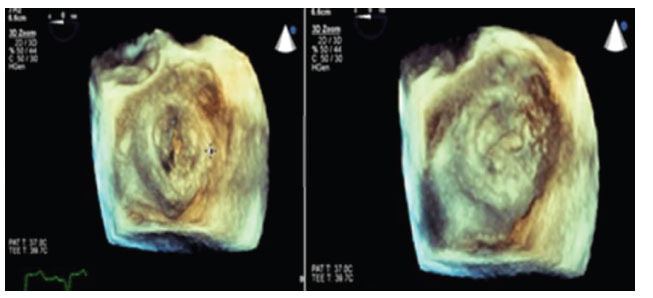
3D Transesophageal echocardiogram showing bioprosthetic mitral valve with multiple large bulky vegetations and dehiscence.
